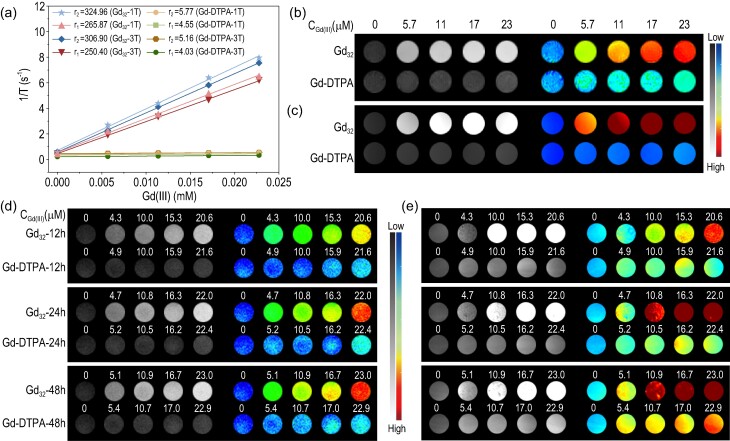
(a) The *r*_1_ and *r*_2_ relaxivities of Gd_32_ and Gd-DTPA solutions containing the same Gd(III) ion concentrations at 1 T and 3 T magnetic fields. Corresponding *T*_1_-weighted MR imaging of Gd(III) ions (Gd_32_ and Gd-DTPA) at 1 T (b) and 3 T (c). *T*_1_-weighted MR imaging of Gd(III) ions (Gd_32_ and Gd-DTPA) incubated with 4T1 cells for different times at 1 T (d) and 3 T (e).
